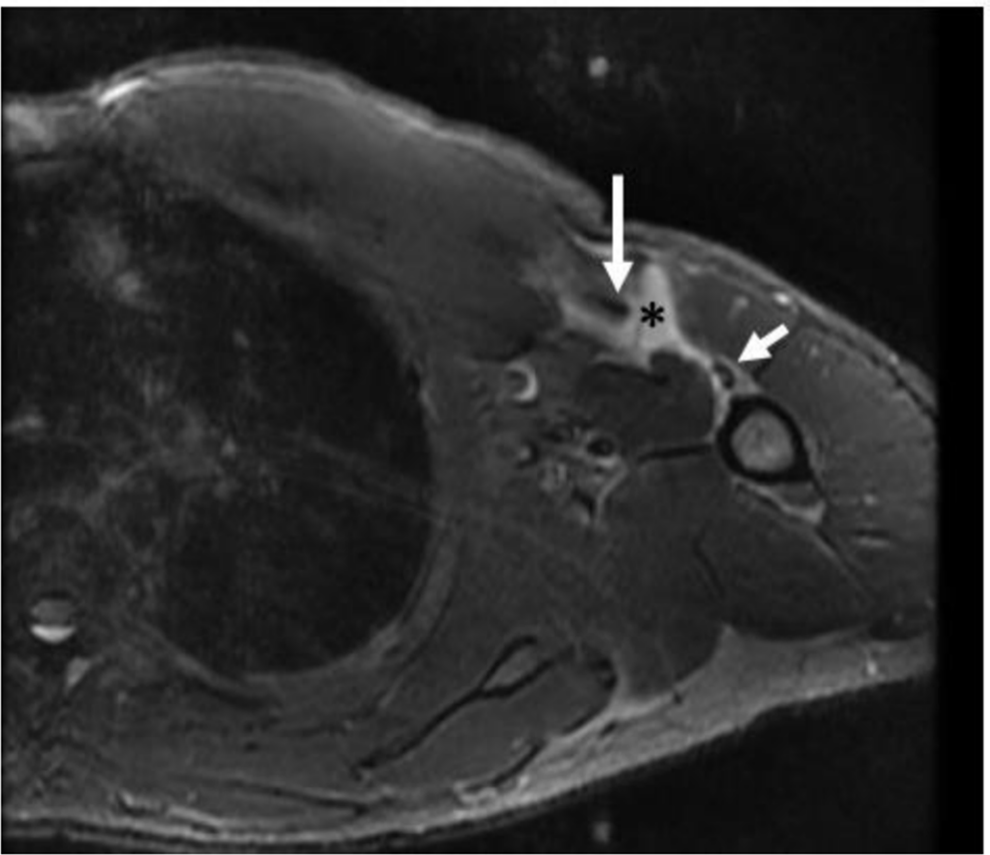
24-year-old-male that presented after feeling a painful pulling and popping sensation while bench-pressing. Axial inversion recovery MR image shows avulsion type complete (full thickness full-width) tear of the pectoralis major tendon from its humeral attachment. The avulsed tendon (long arrow) is medially retracted and there is a small amount of fluid (asterisk) laterally. Note mild anterior displacement of the long head of the biceps tendon. (short arrow). At surgery, there was a complete tear of the tendon from its humeral attachment
